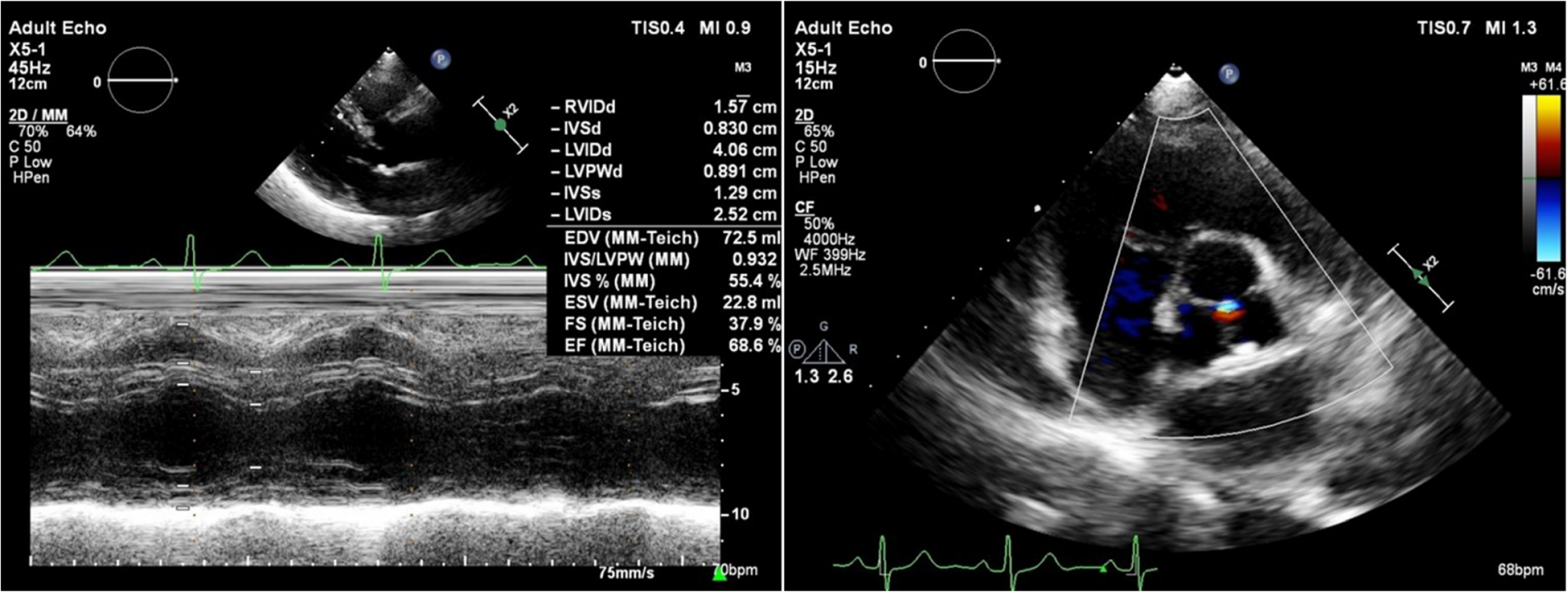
Echocardiogram at 3 months follow-up, showing normal systolic function
